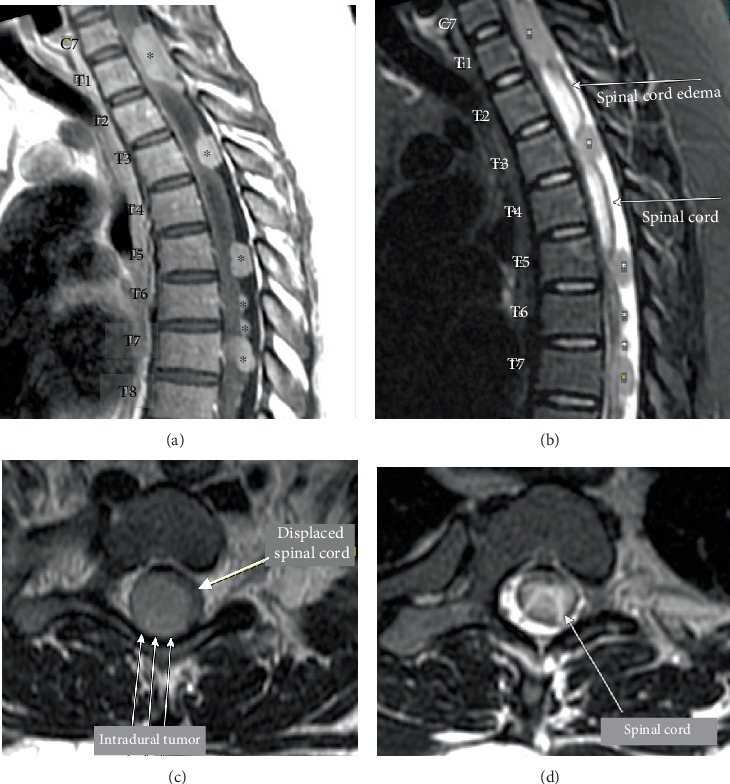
Preoperative images. Preoperative (a) sagittal T1 with contrast and (b) sagittal T2 demonstrating multiple compressive tumors (⁣^∗^) throughout the lower cervical and thoracic spine. Preoperative T2 axial MRI demonstrating an (c) intradural tumor with displaced spinal cord and (d) spinal cord edema.
